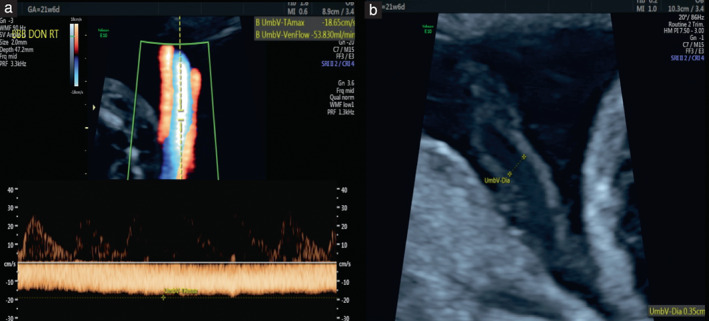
Sonographic method of measuring umbilical vein time‐averaged maximum velocity (TAMX) and diameter (UVD) at a free loop of the umbilical vein. (a) Pulsed‐Doppler waveform showing TAMX measurement of 18.65 cm/s. (b) Adequately zoomed longitudinal section of the umbilical vein with UVD measurement of 0.35 cm.
